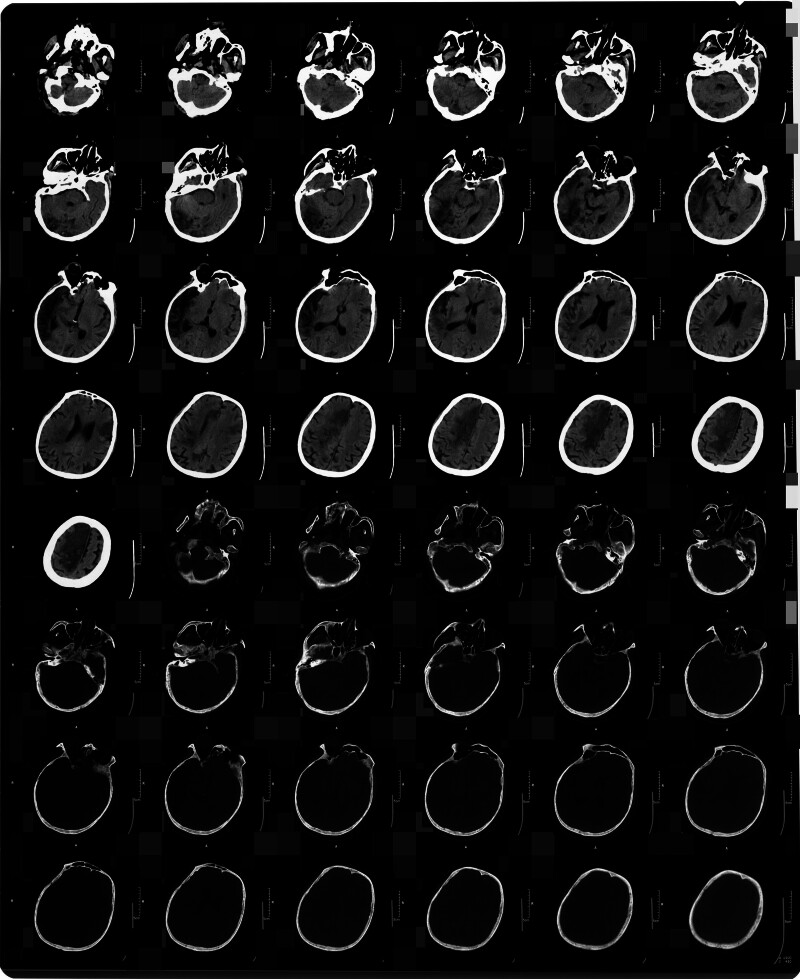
Cranial CT imaging demonstrated irregular hypoattenuating lesions in the right temporoparietal region and bilateral fronto-occipital areas on July 3. CT = computed tomography.
